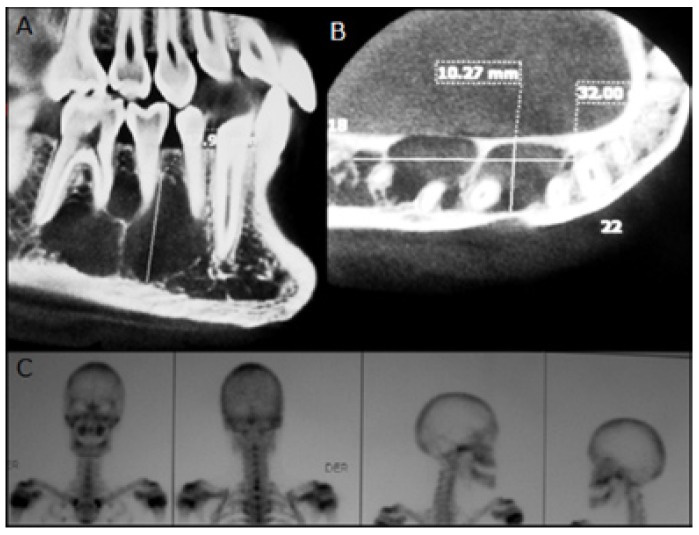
A-B. CB sagittal and axial view. C. bone scintigram.
